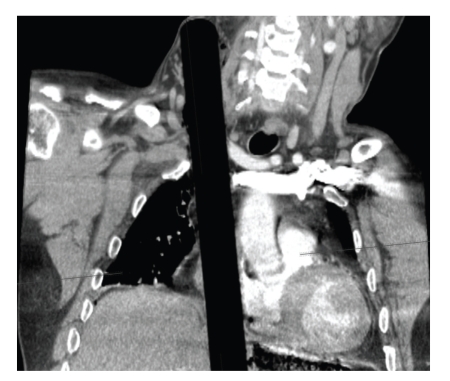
Coronal CT radiograph showing the blunt end of the object extending into the neck.
